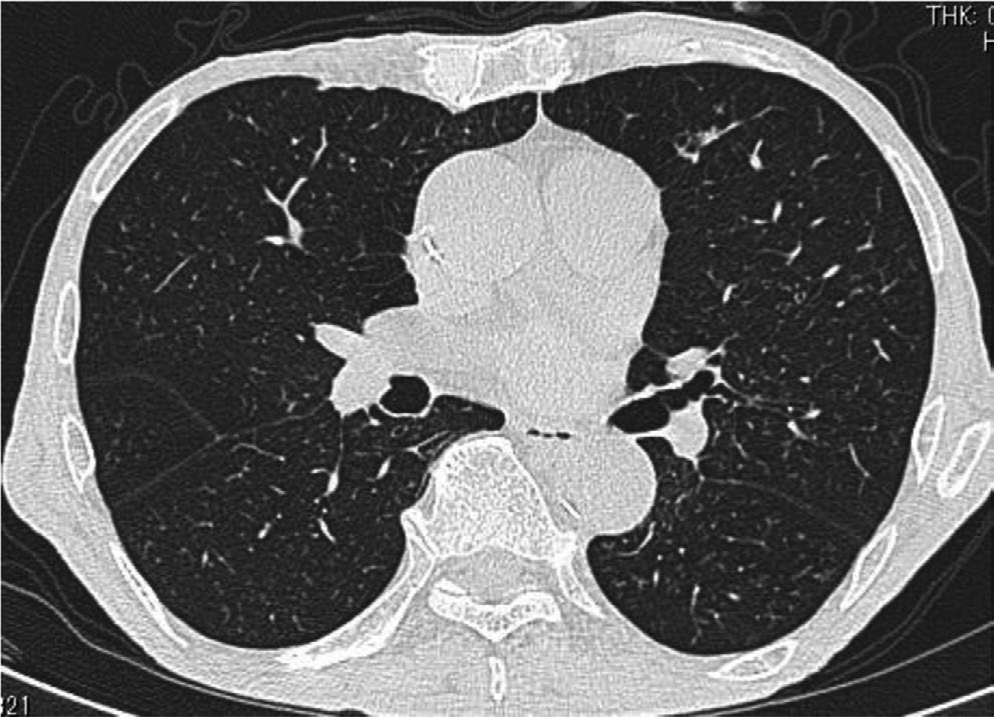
Computed tomography 1 month after hospital discharge shows that many centrilobular nodules with “tree‐in‐bud” branching opacities have disappeared
